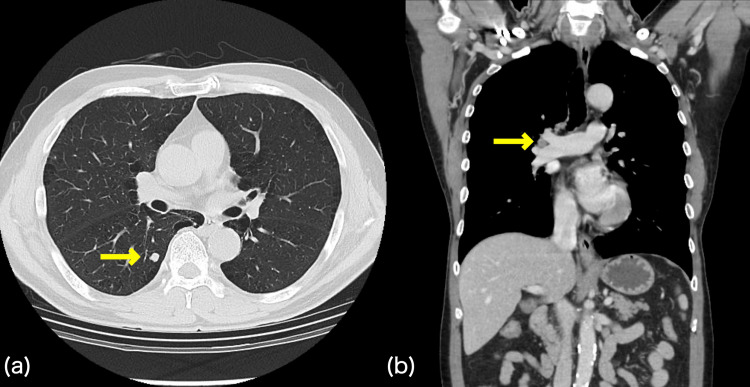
Chest computed tomography scan at the time of tumor detection (a) Axial view shows prominent emphysematous changes. A nodule sized 8 mm was found in S6 of the lower lobe of the right lung. There was no obvious calcification and no surrounding nodule or granular shadow. (b) Coronal view demonstrates small right hilum lymphadenopathy. (a, b) Based on the two findings, the patient was considered in clinical stage IIB (T1a, N1, M0).
